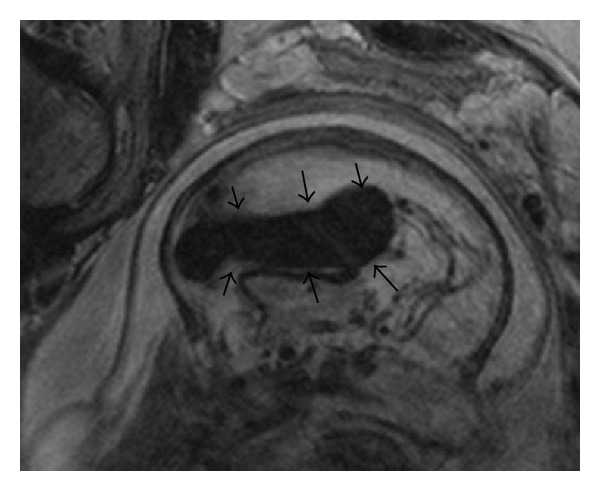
Fetal MRI imaging of aneurysm of vein of Galen.
